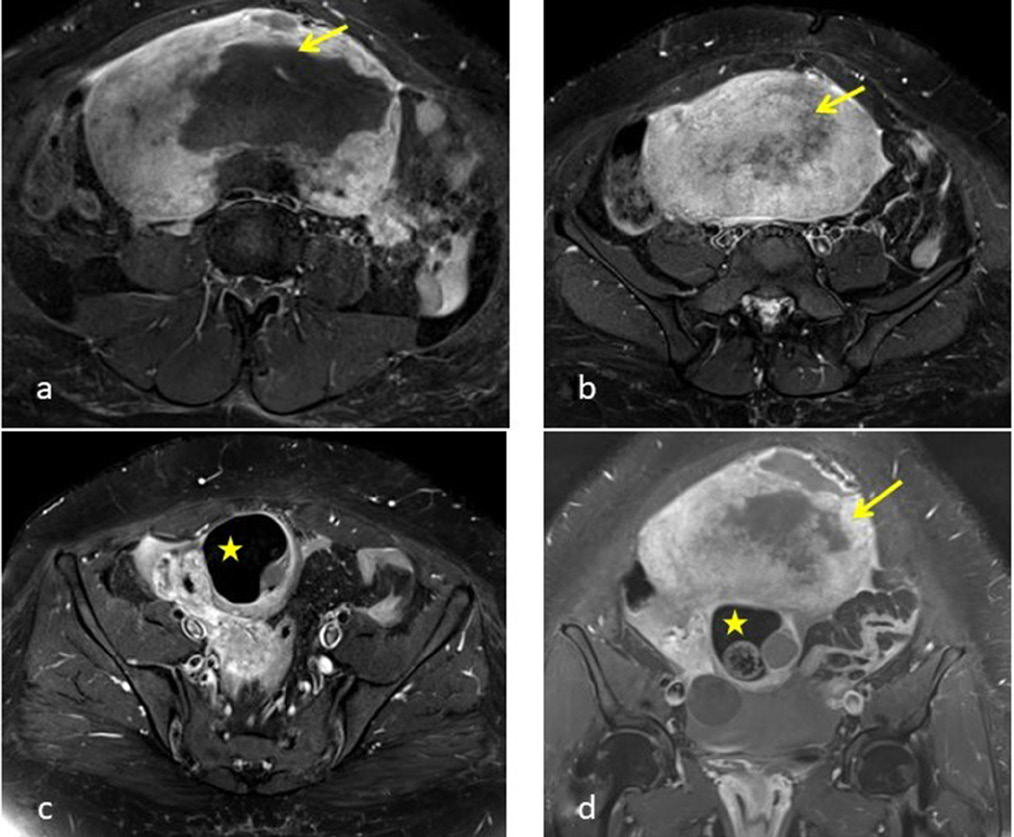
(a, b): MRI Axial T1 and T1 FS showing fat suppression within
